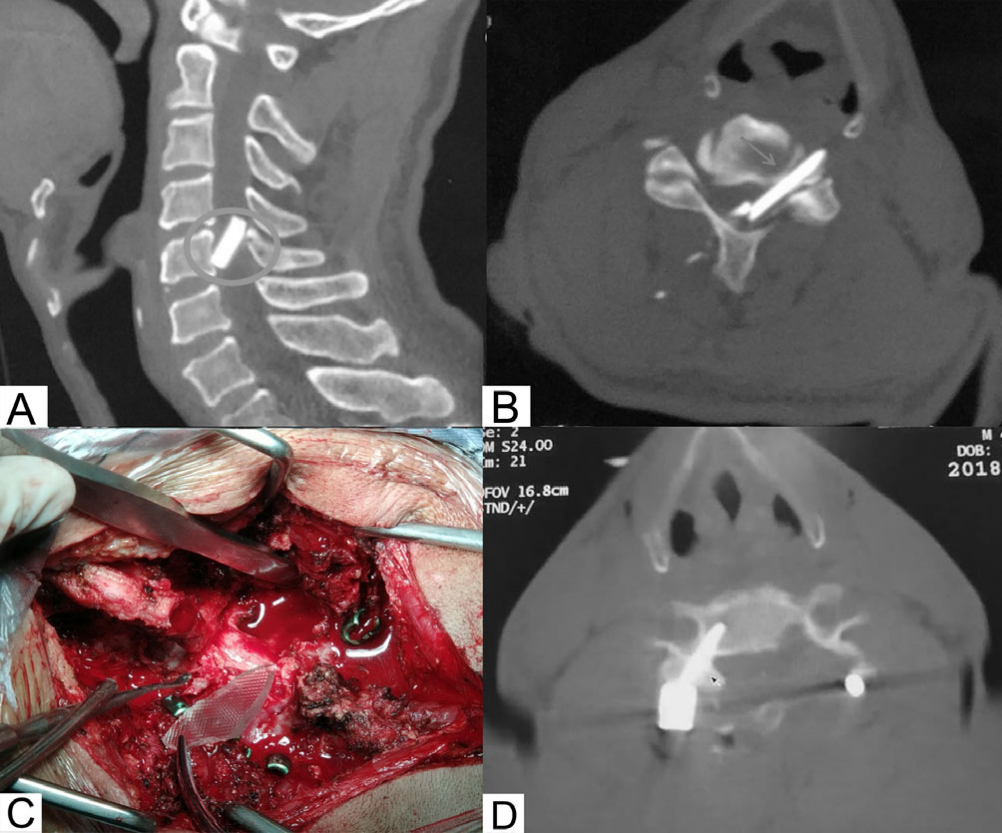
Case 2 (49‐year‐old male). (A) and (B) CT of the cervical spine indicated that foreign glass entered the spinal canal at the level of C_4_; (C) after removing the foreign glass, no more hemorrhage was induced and (D) laminectomy and internal fixation with lateral mass screws
